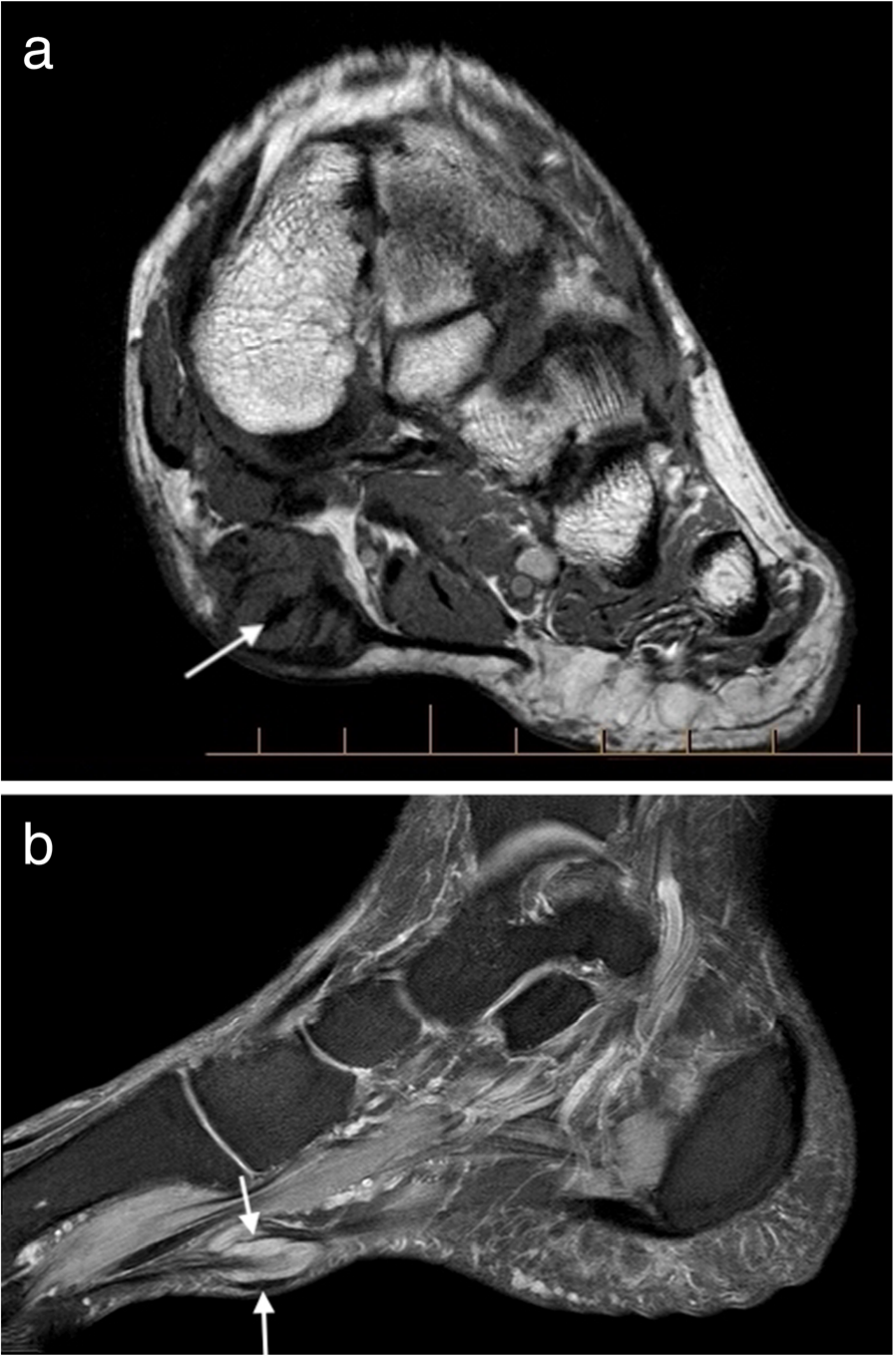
Plantar fibroma. a Sagittal T2 fat suppressed (T2FS) and (b) coronal T1-weighted image of the foot demonstrating a heterogeneous mass centred on the plantar fascia, distinct from the underlying musculature. The characteristic low signal linear bands are shown (arrows)
